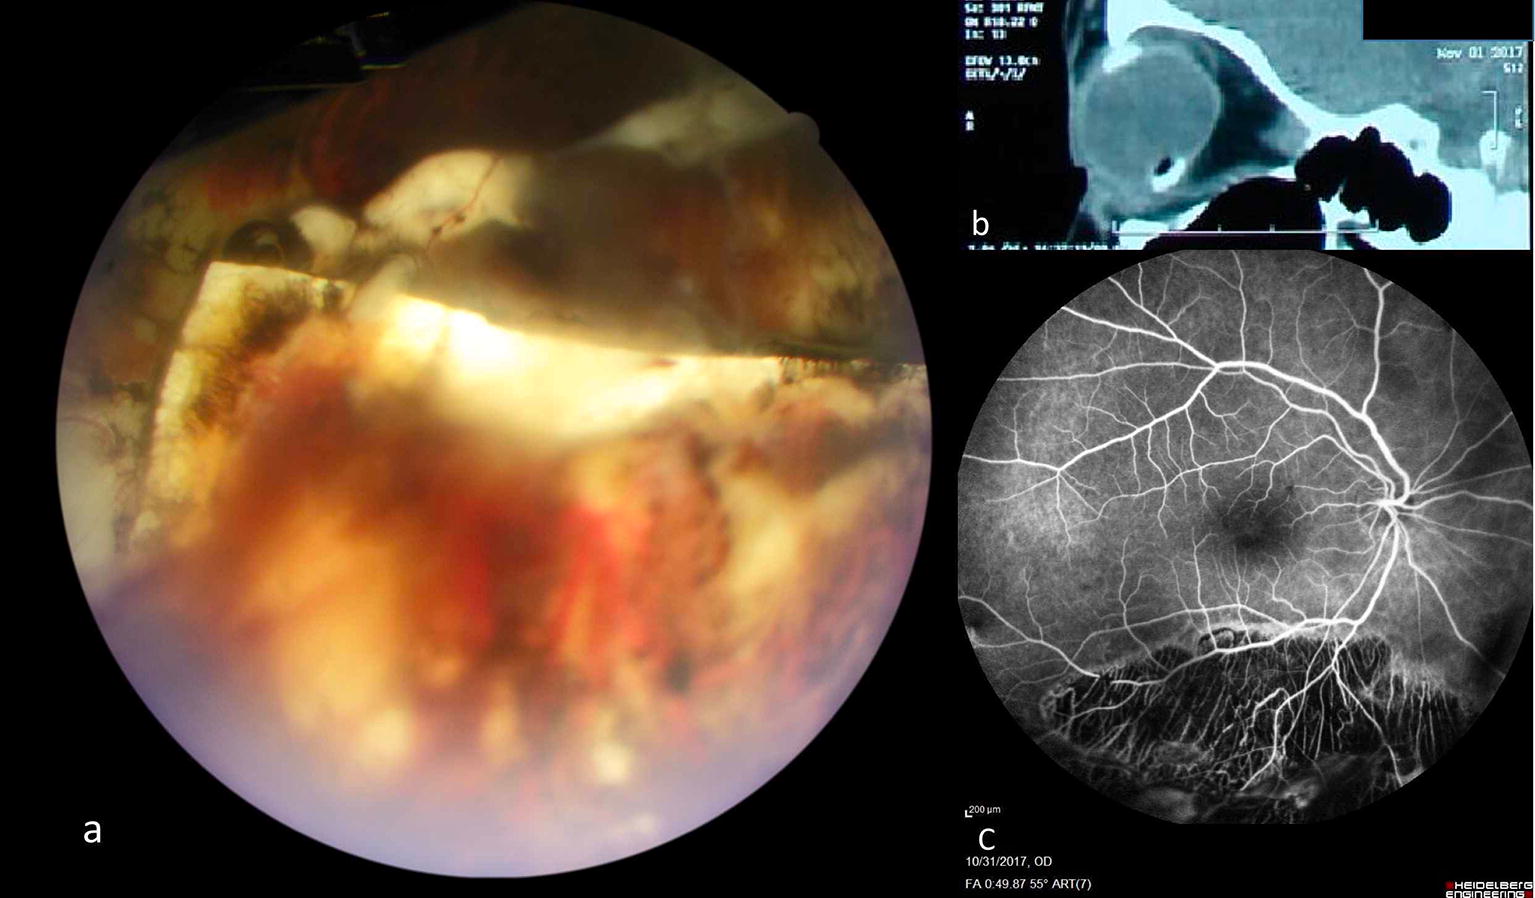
Case 1: a An intruded silicone sponge, partially covered with blood is seen in inferior vitreous cavity. Adjacent retina and choroid show extensive pigmentary and atrophic changes. b In sagittal orbital computed tomography (CT) scan, intruded hypodense silicone sponge is seen over the hyper dense silicone rubber (tire and band complex) in inferior globe. c Fluorescein angiography shows an extensive hypofluorescent area caused by severe chorioretinal atrophy in inferior retina
